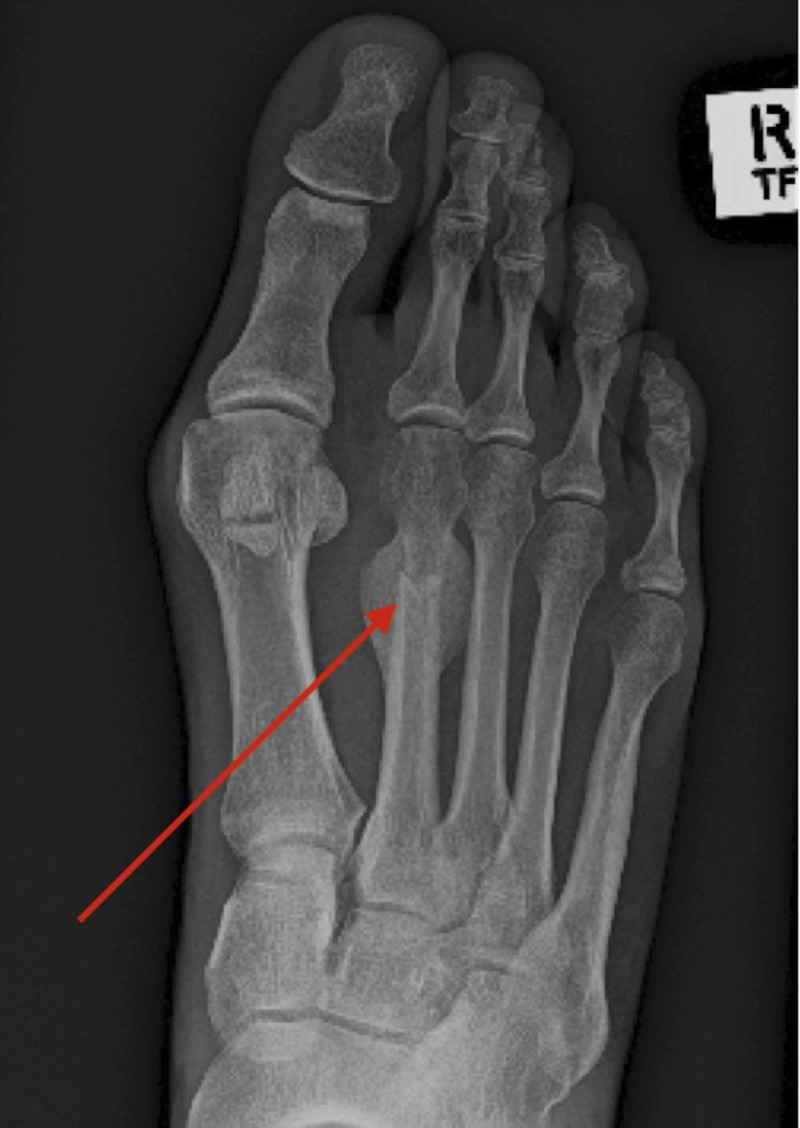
Anterior-Posterior radiograph showing the metatarsal fracture with osteophytic bone formation
